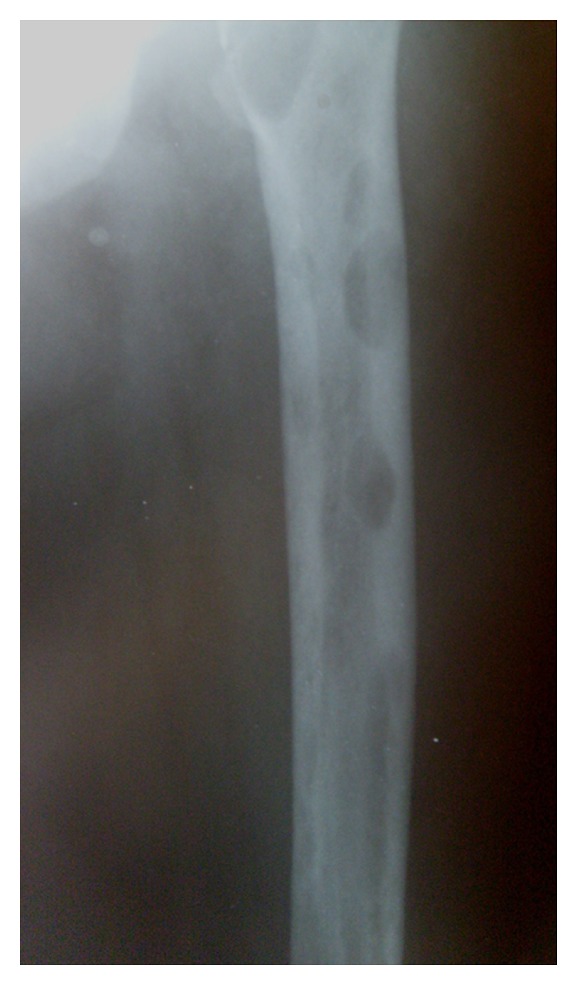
Radiograph of the left femur showing multiple lytic lesions.
